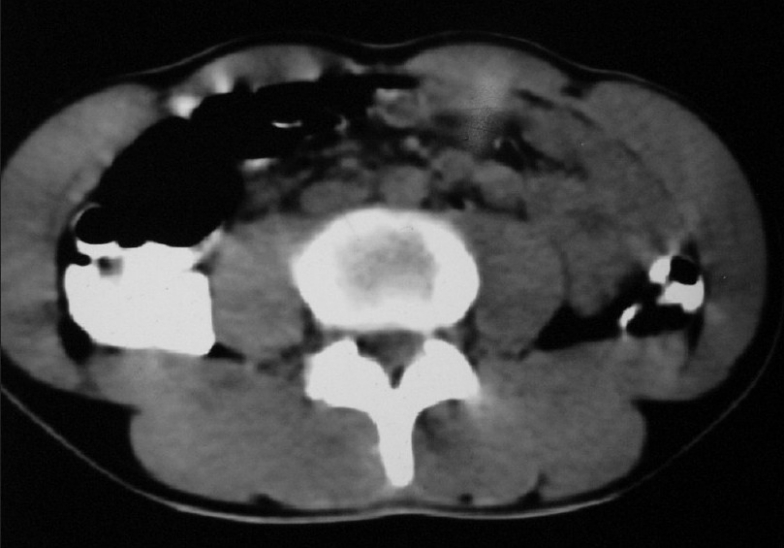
CT abdomen (2007) shows multiple para aortic lymphadenopathy
